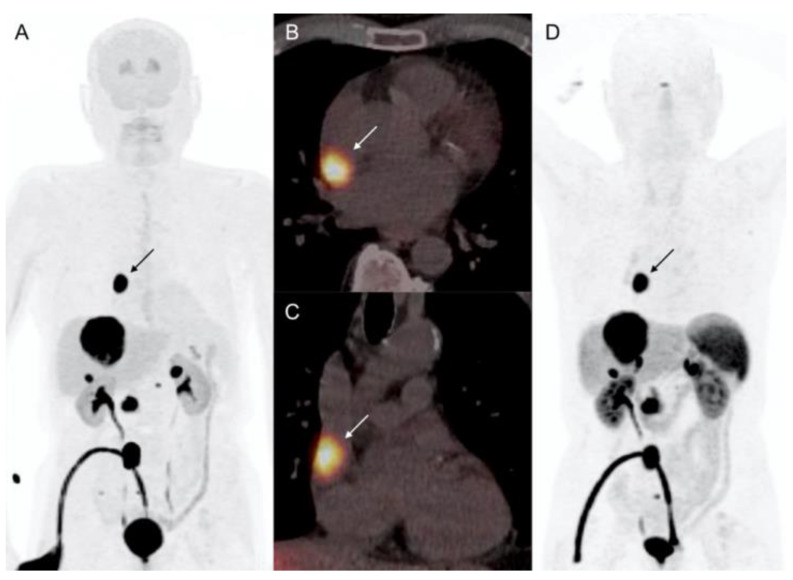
Patient 4. 76-year-old patient with metastatic si-NET. LV CM (arrows) showed intense uptake of both ^18^F-DOPA and ^68^Ga-DOTATOC. (A) ^18^F-FDOPA PET MIP (anterior projection). (B,C) ^18^F-FDOPA PET/CT short axis, and 4 chamber view. (D) ^68^Ga-DOTATOC PET MIP (anterior projection).
